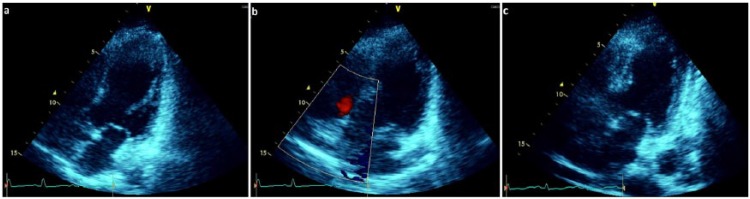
(a) Transthoracic echocardiogram (TTE) parasternal long-axis view revealing severe left ventricular function of 20% to 25% with apical ballooning and spontaneous “echo-smoke.” (b) TTE apical 2-chamber view showing apical ballooning. (c) TTE apical 2-chamber off-axis view showing apical ballooning and dyskinesis.
